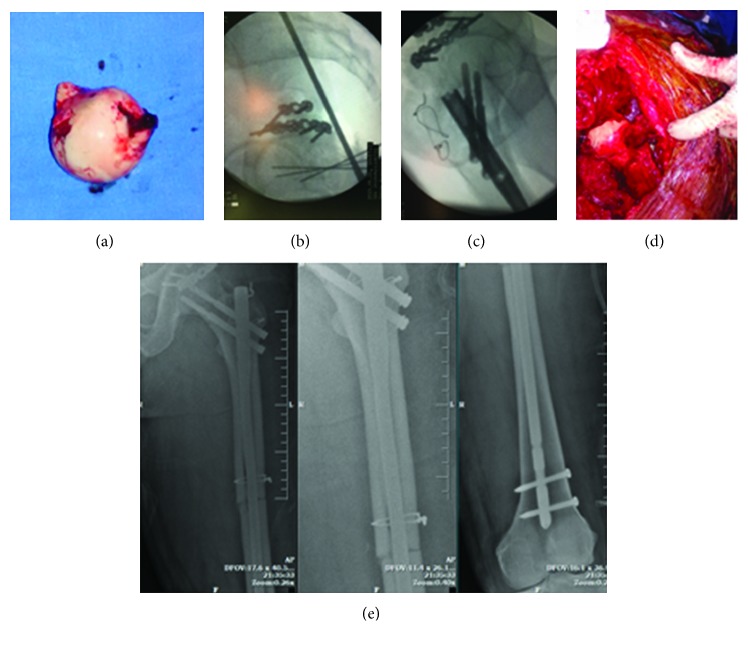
Intraoperative images of the displaced femoral head (a) which was reduced and fixed following the acetabulum fixation (b, c, d). Postoperative radiograph (e).
